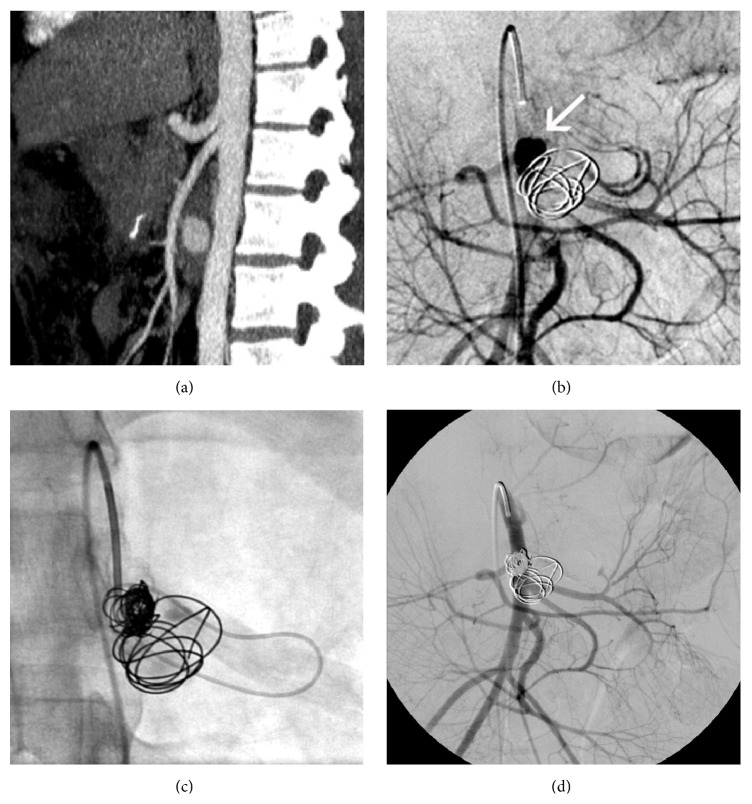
34-year-old woman presenting a jejunal artery pseudoaneurysm treated by transcatheter embolization via collaterals vessels. (a) Sagittal contrast enhanced CT image showing a pseudoaneurysm emerging from the first SMA branch (jejunal artery) just after its origin. (b) Digital angiogram obtained after coil embolization shows that pseudoaneurysm is still partially patent (white arrow) requiring further embolization; however subsequent direct catheterization was not possible. The image shows a collateral vascular arc between the first and the second jejunal arteries. (c) The collateral vascular arc was used to obtain pseudoaneurysm catheterization. (d) Complete embolization was finally obtained.
